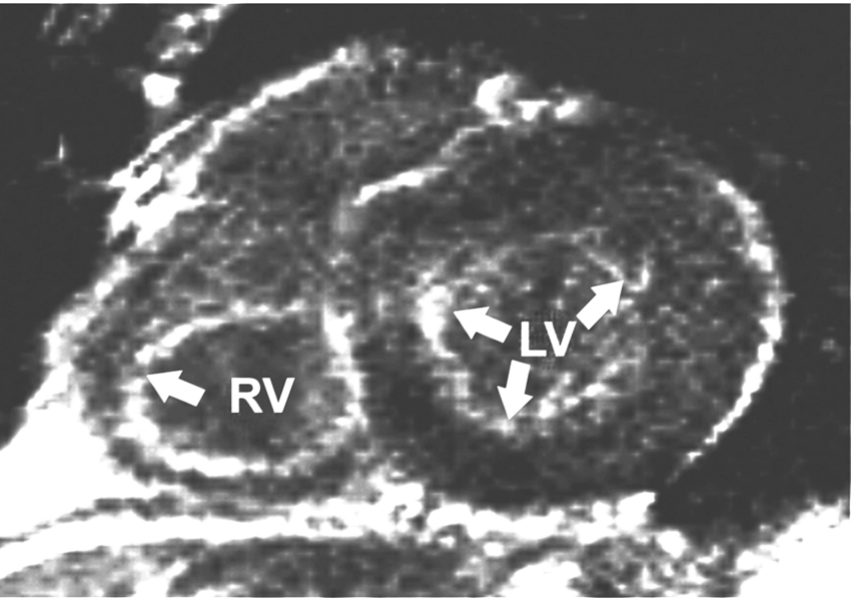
Magnetic resonance imaging of patient number 1 (short axis) showing a diffuse signal enhancement (arrows) of the left ventricular myocardium (late enhancement, segmental inversion recovery - TurboFLASH 2D image). RV: right ventricle. LV: left ventricle.
